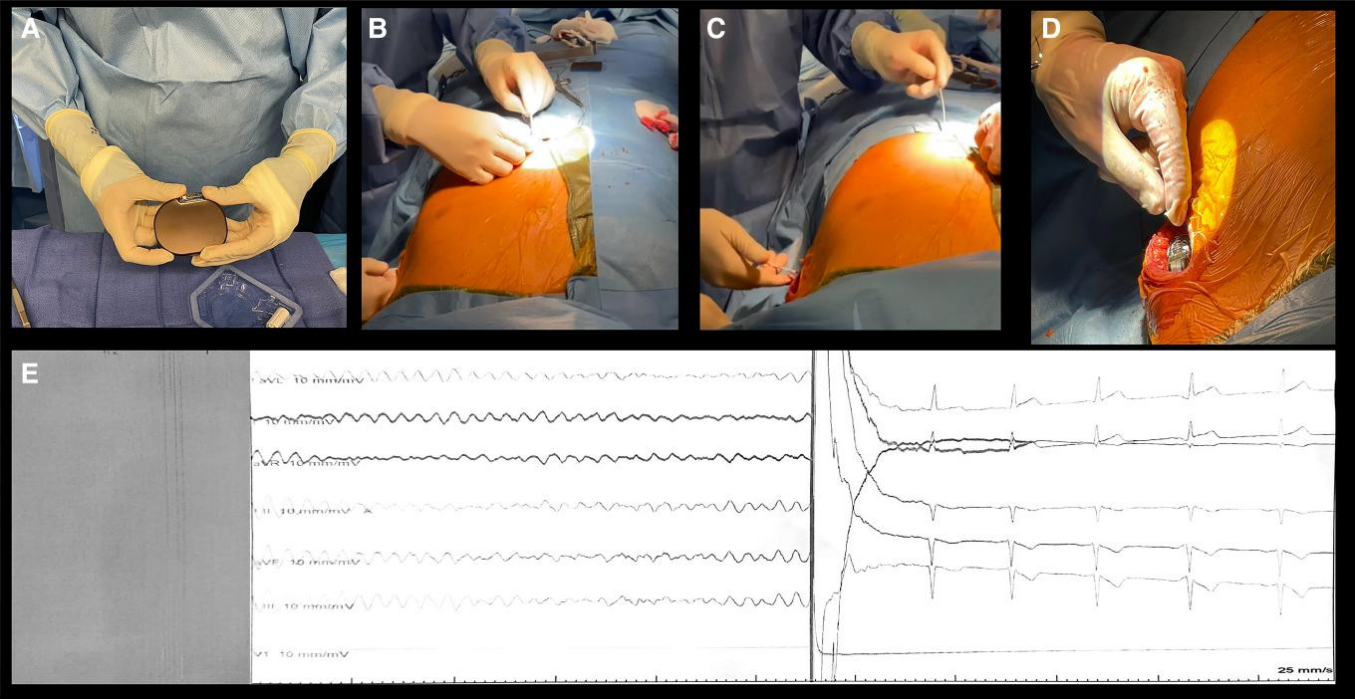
Sequence of subcutaneous implantable cardioverter-defibrillator placement (*A–D*) X-ray showing proper electrode and device position. (*E*) The induced ventricular fibrillation is terminated and sinus rhythm is restored with a shock delivered by the subcutaneous defibrillator.
